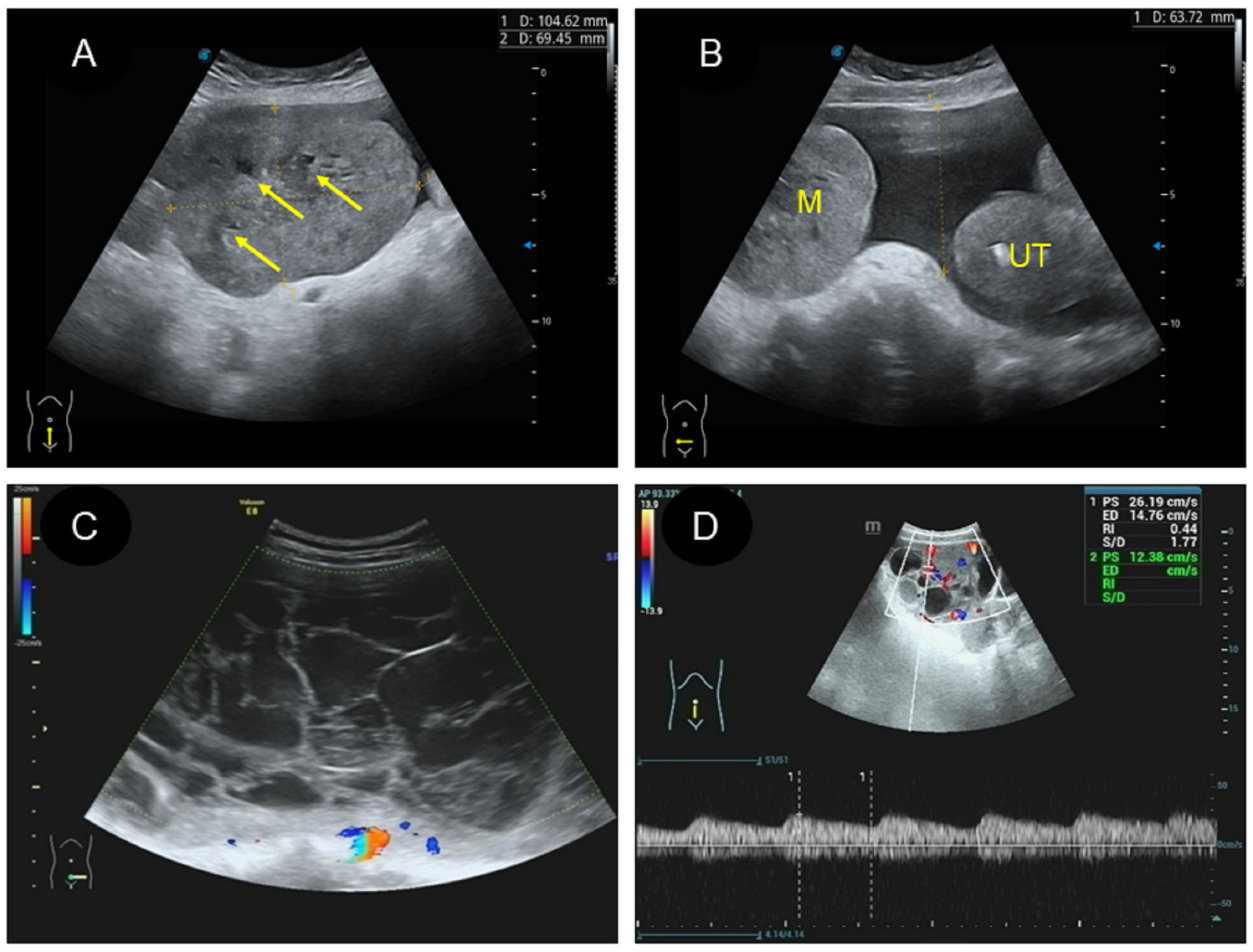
Some specific ultrasound findings of different types of yolk sac tumor. (A).” Fissure sign “ inside the solid yolk sac tumor (thin arrow); (B). A solid yolk sac tumor complicated with a large amount of ascites; (C). A large number of irregular arrangement separation in cystic type of yolk sac tumor, and CDFI showed no blood flow signal in separation; (D). PW showed low resistance of artery in the solid type of yolk sac tumor.
